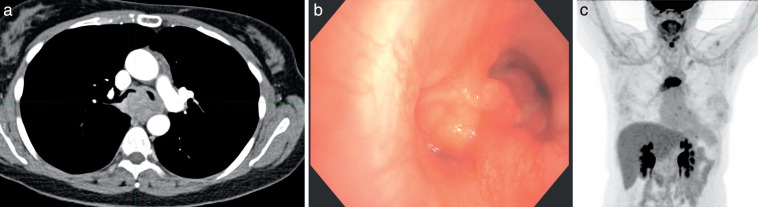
Initial evaluation of the adenoid cystic carcinoma on (a) contrast CT scan, (b) bronchoscopy, and (c) FDG-PET scan. Abbreviations: CT, computed tomography; FDG-PET, fluorodeoxyglucose positron emission tomography.
